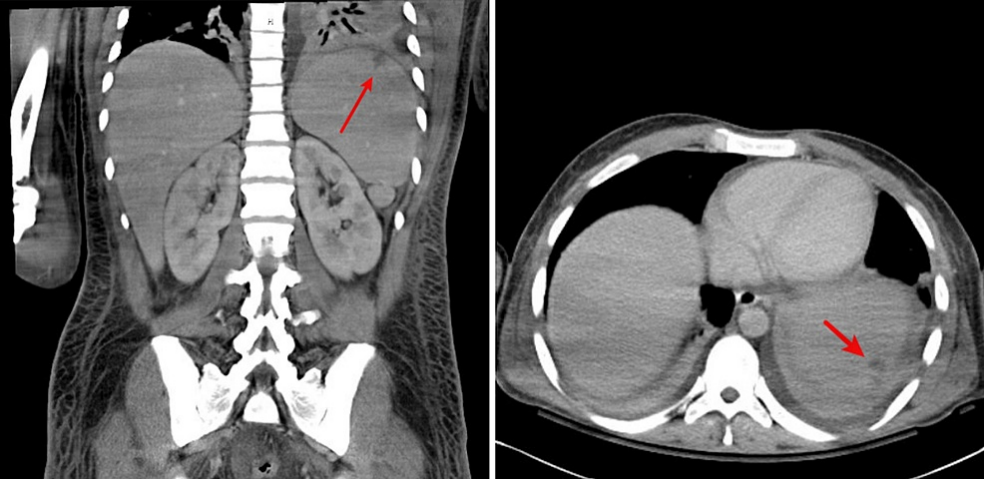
CT scan of the abdomen exhibits a small wedge-shaped (indicated by the arrow) peripheral splenic infarct. CT: computed tomography.
